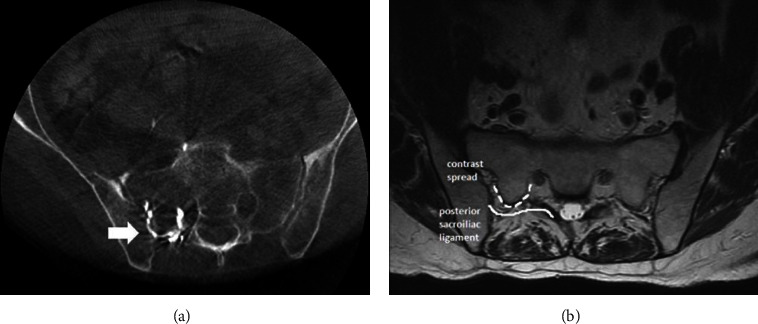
3D-CBCT images (a)—Arrow indicating contrast spread in the right sacroiliac joint tracking into the right posterior S1 neuroforamen. The similar level axial plane on the lumbar spine MRI (b) demonstrating posterior sacroiliac ligament (solid line) which corresponds to this contrast track (dotted line indicating the track through which contrast spreads from the sacroiliac joint into posterior S1 neuroforamen).
